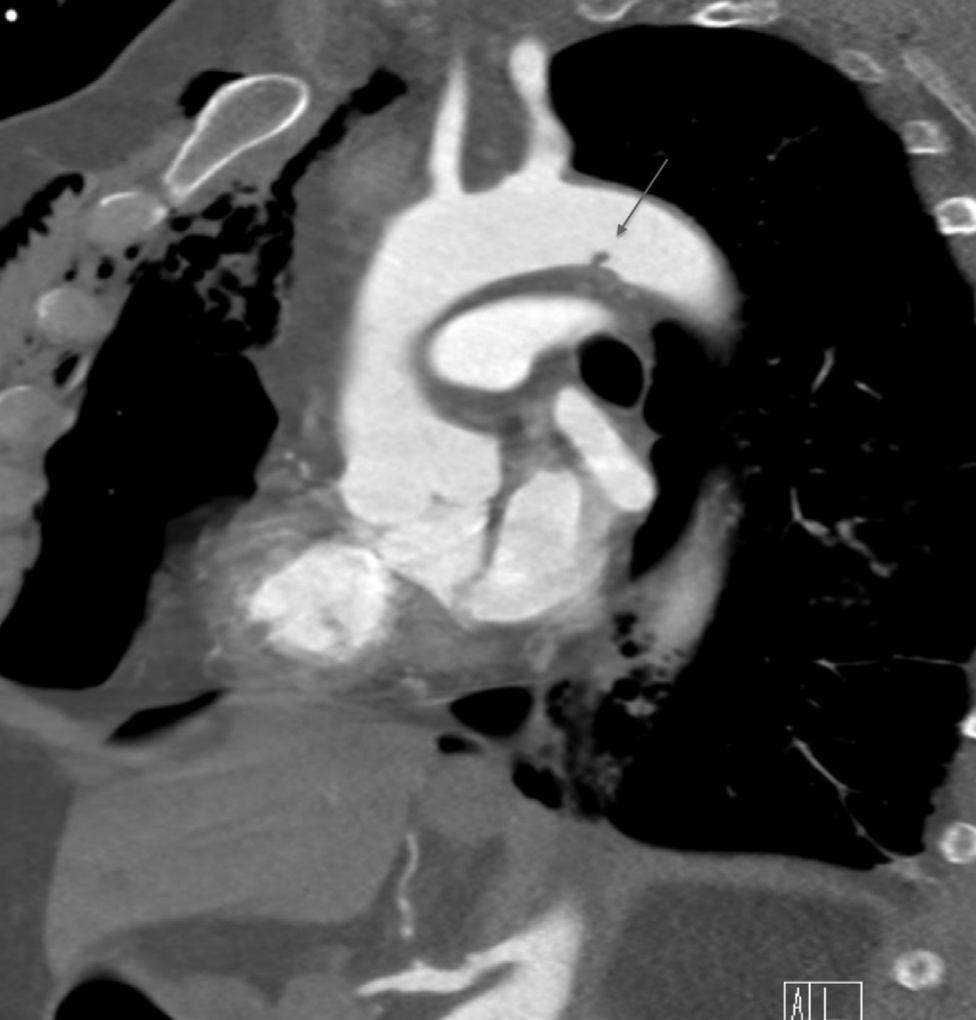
Contrast-enhanced sagittal oblique computed tomography angiography. (mediastinal windows) shows a <1.0 cm intimal flap, grade I injury, along the inferior aspect of the aortic arch (gray arrow). Note the hemopneumomediastinum and anterior chest wall subcutaneous air. This injury demonstrated complete healing on follow-up CTA imaging in 7-days' time.
